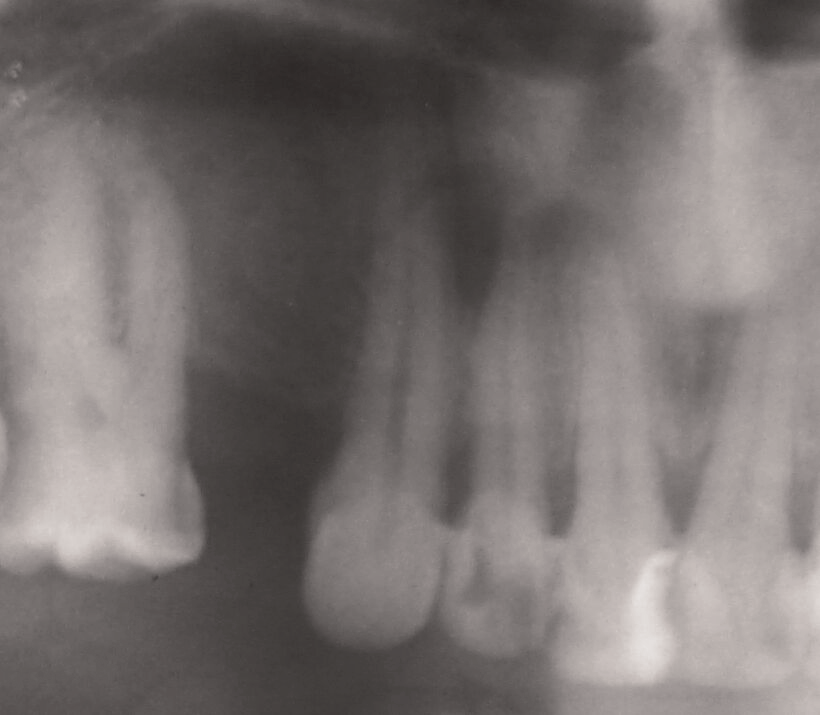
Dental panoramic tomography showing periapical lesion associated with upper right lateral incisor.
